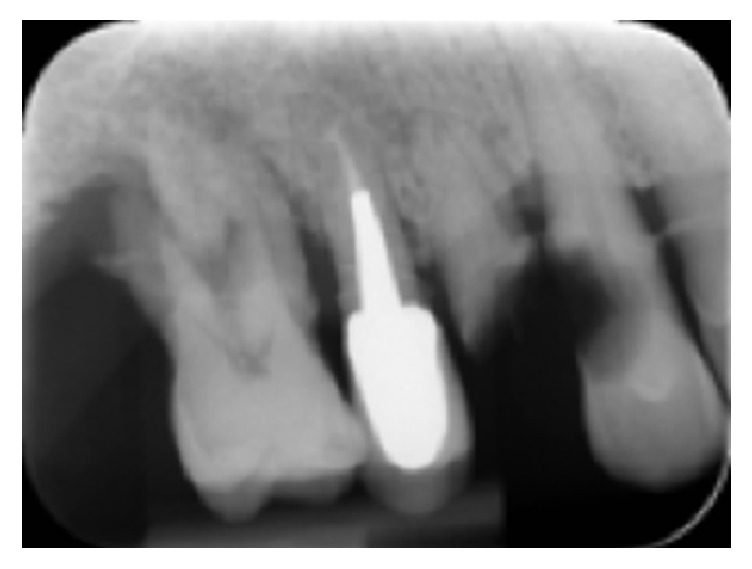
Presurgical radiographic view.
